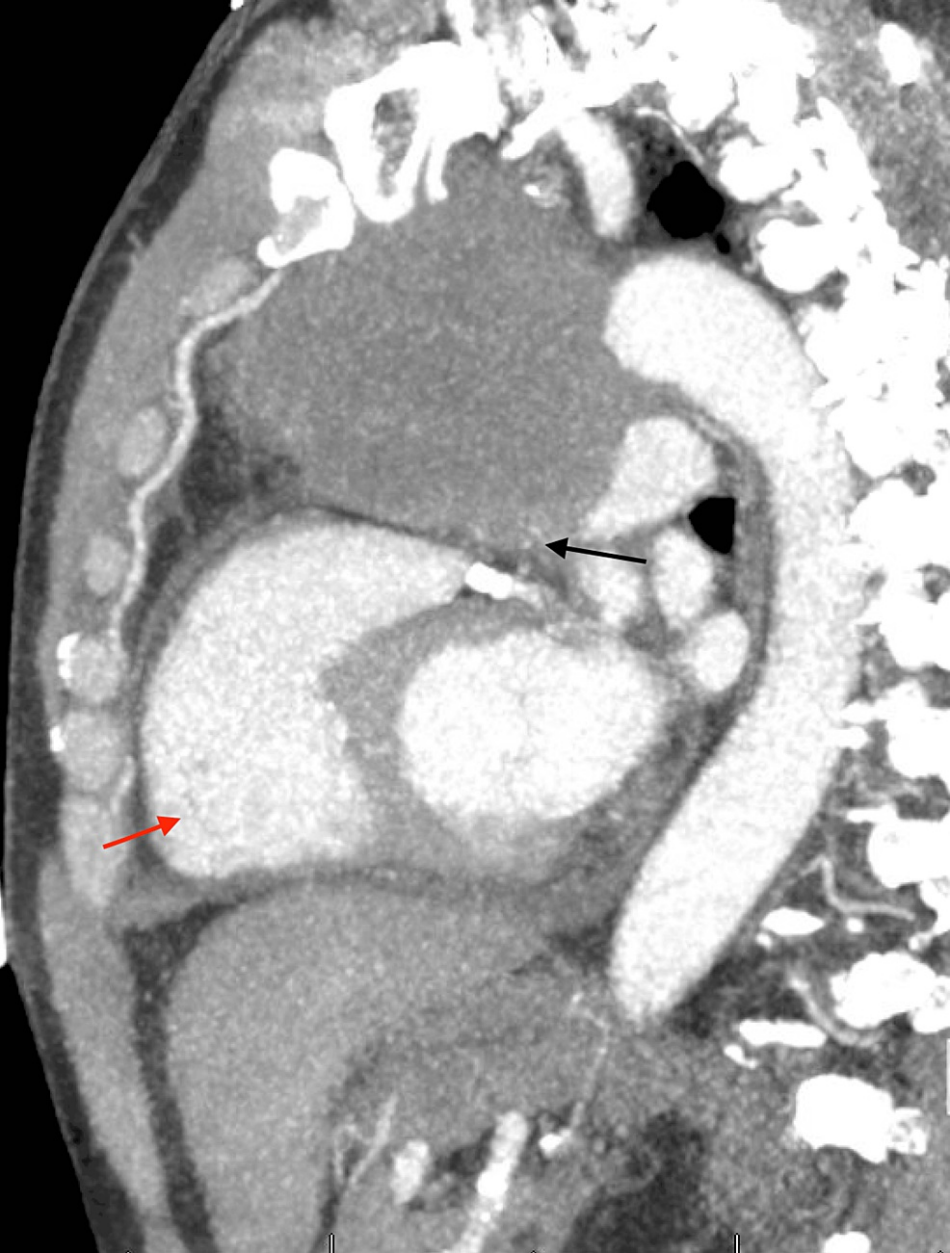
Chest CT post-pericardiocentesis CT with intravenous contrast mid-sagittal view showing large anterior mediastinal mass with significant main pulmonary artery compression (black arrow). There is evidence of right heart strain with an RV-to-LV ratio of approximately 1.5 (red arrow) CT: computed tomography; LV: left ventricle; RV: right ventricle
